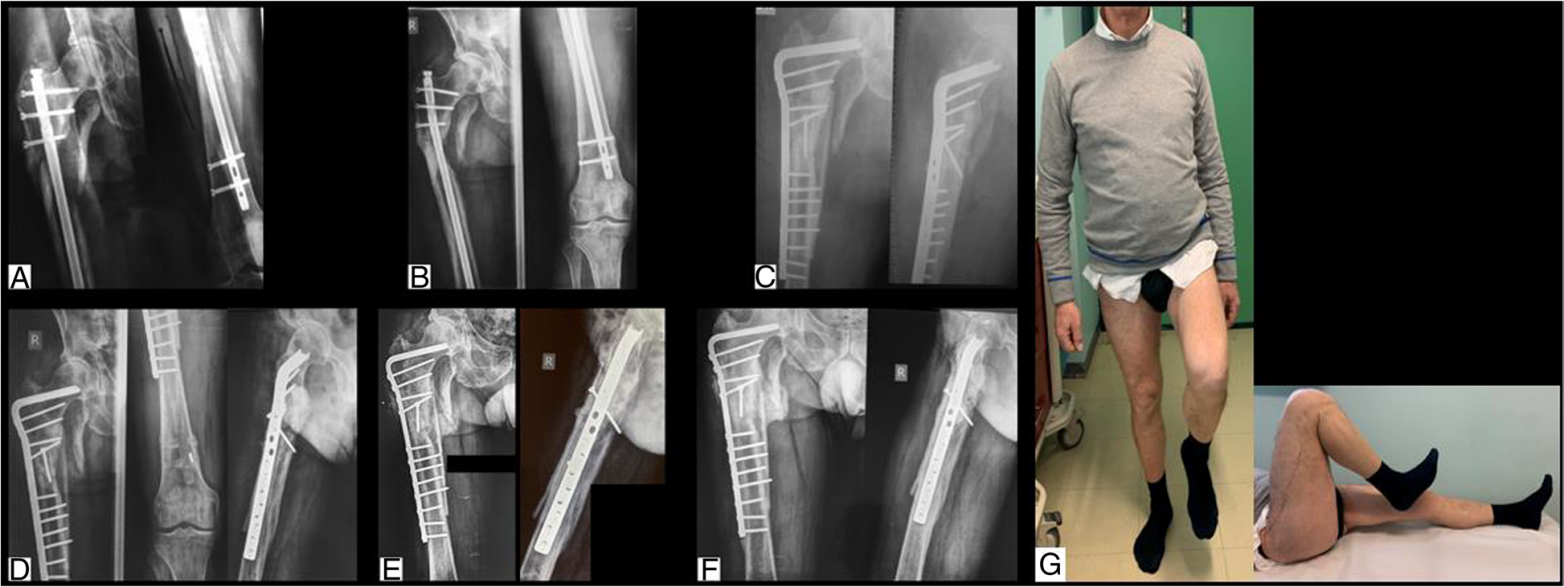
A radiograph of femur subtrochanteric fracture of a 55 years old man, following a high-energy trauma; B radiographs of the non-union with a broken EM nail, at 5 months post-op; C AP and LL radiographs of the reintervention with 95° blade plate, medial strut allograft, RIA system and lag screw; D AP and LL radiographs at 2 months follow-up; E AP and LL radiograph at 4 months follow-up; F AP and LL radiograph at 9 months follow-up; G clinical evaluation of the patient at 9 months follow-up
